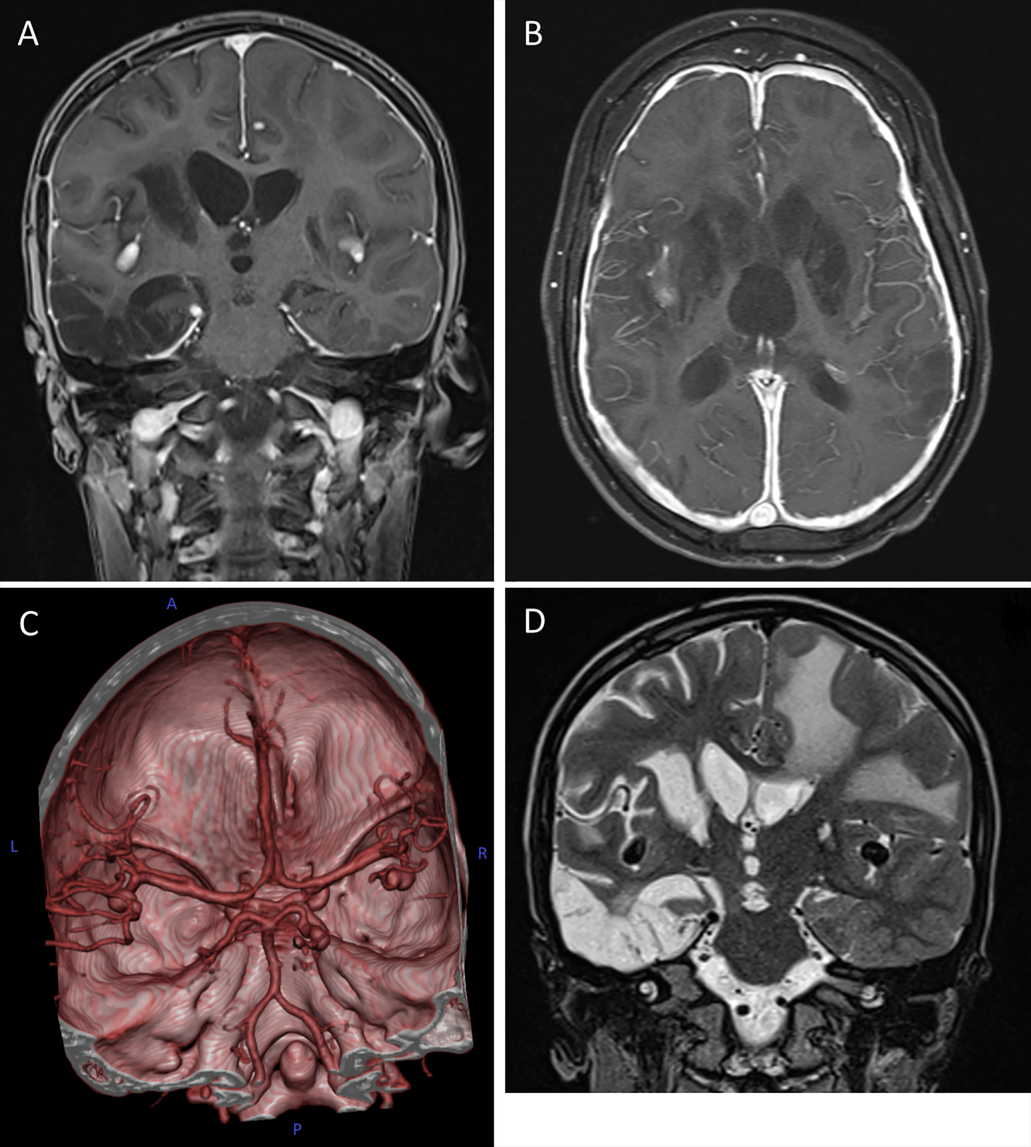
Cerebral magnetic resonance imaging (cMRI) of the index patient at age 10. (A, B) T1-weighted, contrast enhanced MRI scans in coronal (A) and axial (B) plane depicting the hypertrophic pachymeningitis with bilateral basal ganglia, midbrain and cortical lesions. Note the postinfectious internal hydrocephalus. (C) 3D reconstitution of the vascular system of the brain showing bilateral media aneurysms and a posterior cerebral artery aneurysm on the right without signs of vessel narrowing. (D) T2-weighted MRI scan in coronal plane highlighting the extensive frontotemporal white matter edema on the left, global parenchymal atrophy and the medial cerebral artery aneurysms bilaterally.
